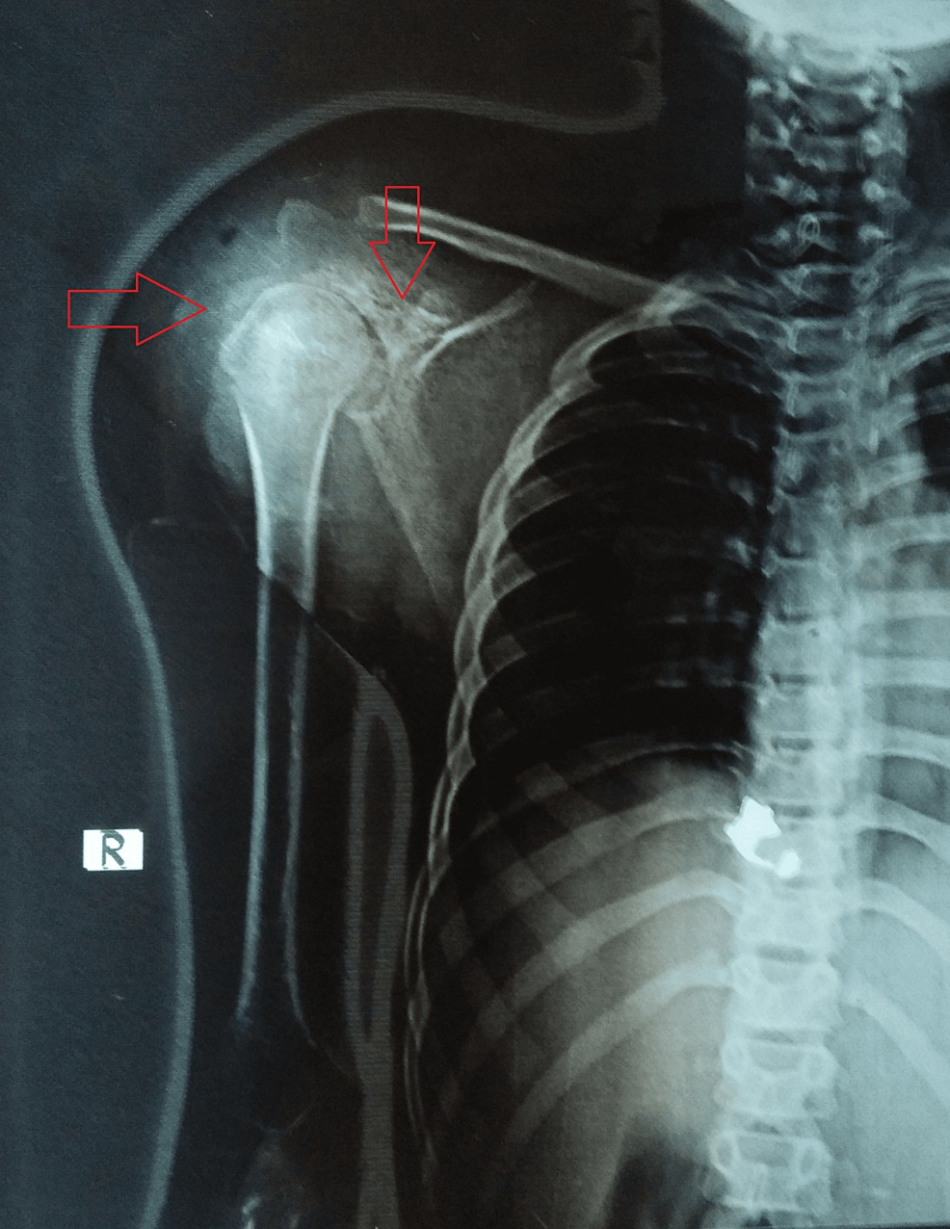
A plain radiograph of the right shoulder suggestive of a soft tissue swelling with erosions of the scapula and osteopenia of the humerus with narrowing of the joint space.
